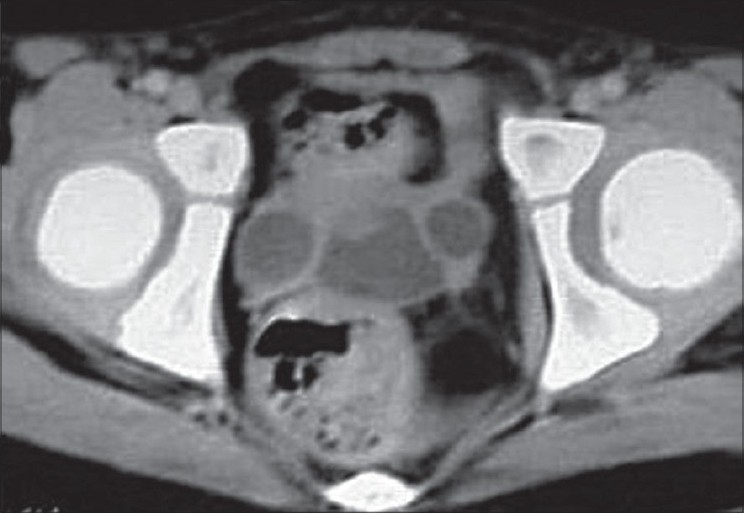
Computed tomography scan: Bilaterally dilated ureters with a small bladder
